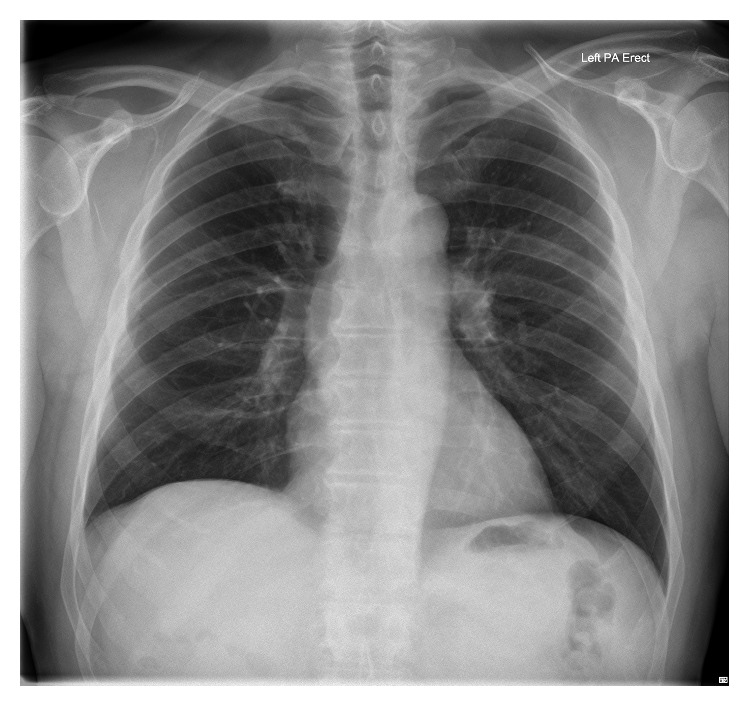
Chest radiograph three months postoperatively showing resolution of right hemidiaphragm paralysis.
